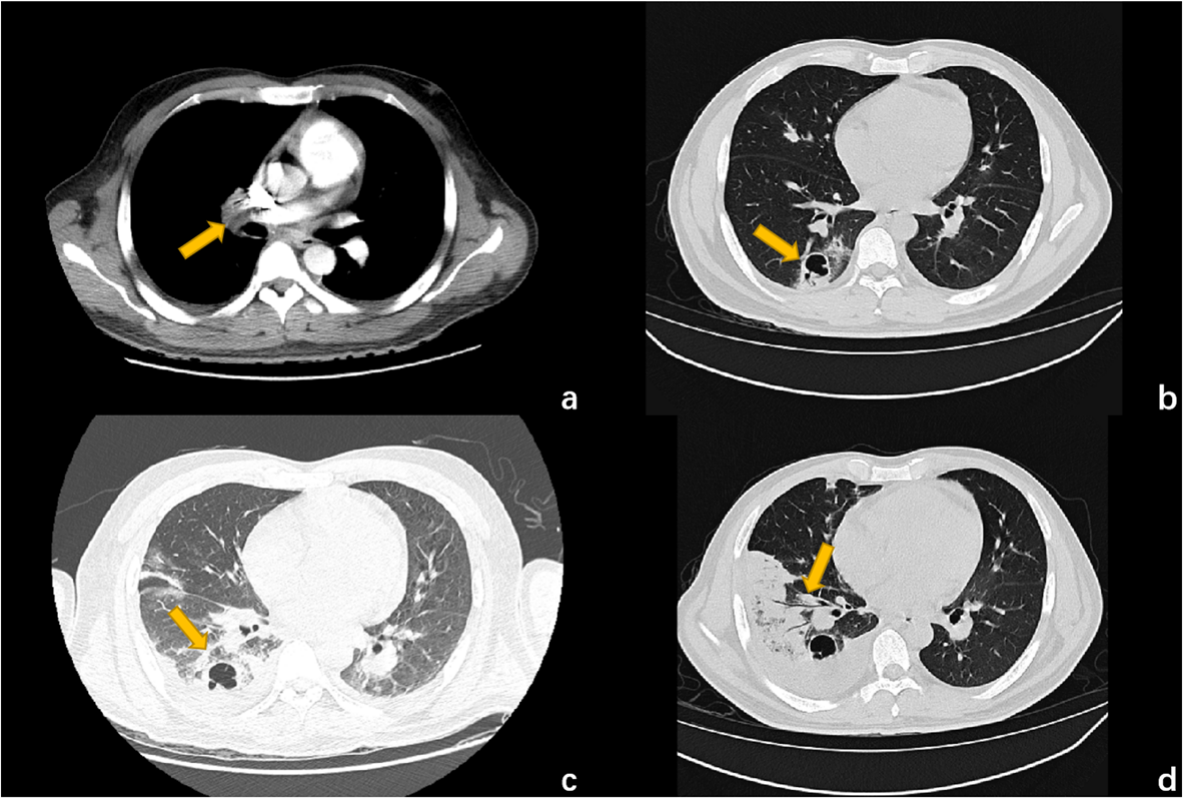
a. Computed tomographic pulmonary angiography on day 2 after admission. The arrow indicates the filling defect of the right pulmonary artery trunk. b-d. Chest computed tomography image on days 1, 5 and 27. Arrows indicate the cavity and inflammatory lesions in the right lung
